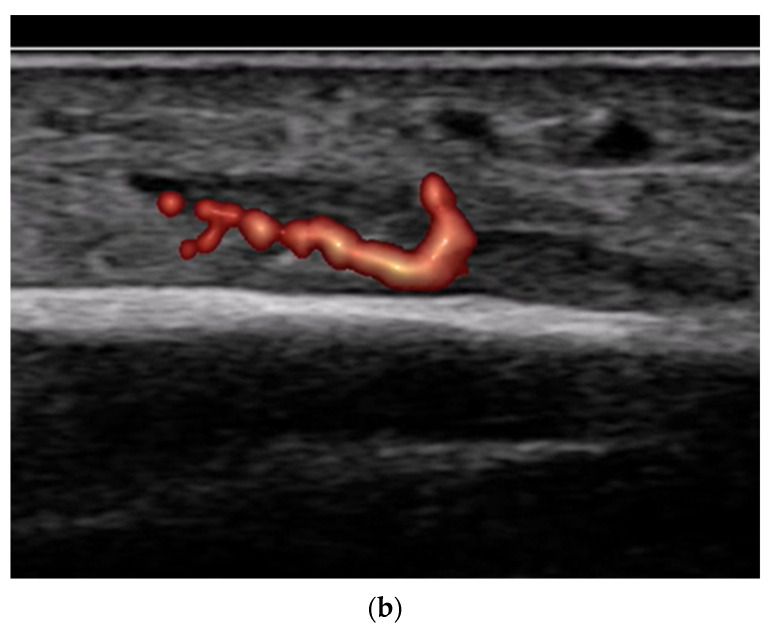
Stress fracture metatarsal. (a) Subtle bone bulge, periosteal reaction and mild subcutaneous edema. (b) Same patient with power Doppler shows inflammatory vessels.
